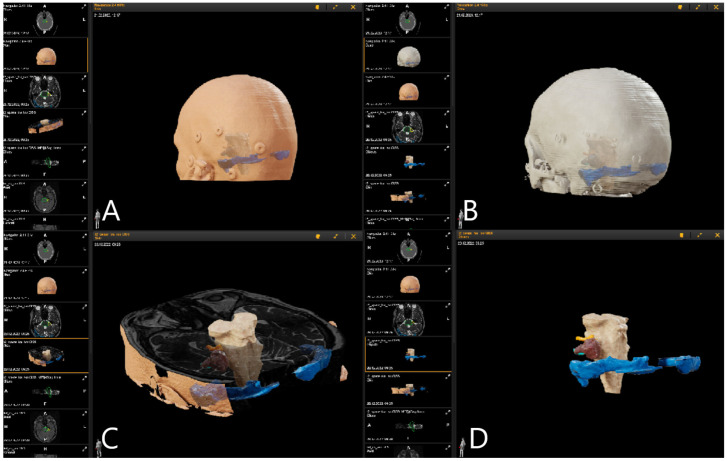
Preoperative approach and resection planning (Patient No. 39). Fusion of preoperative MRI and preoperative navigation-CT was performed. Objects of interest—tumor, trigeminal nerve, transverse and sigmoid sinus—were segmented manually and using autosegmentation with an anatomical mapping element. (A) Skin view for approach planning in sitting position with presentation of segmented objects and fiducials. (B) View of reconstruction of bony structures using the CT. (C) Reconstruction of segmented objects in T2-weighted CISS sequence. (D) Visualization of the tumor (brown), brain stem (white), trigeminal nerve (orange) and transverse and sigmoid sinus (blue) as a 3D object following segmentation in T2-weighted CISS sequence and T1-weighted post-contrast MRI.
